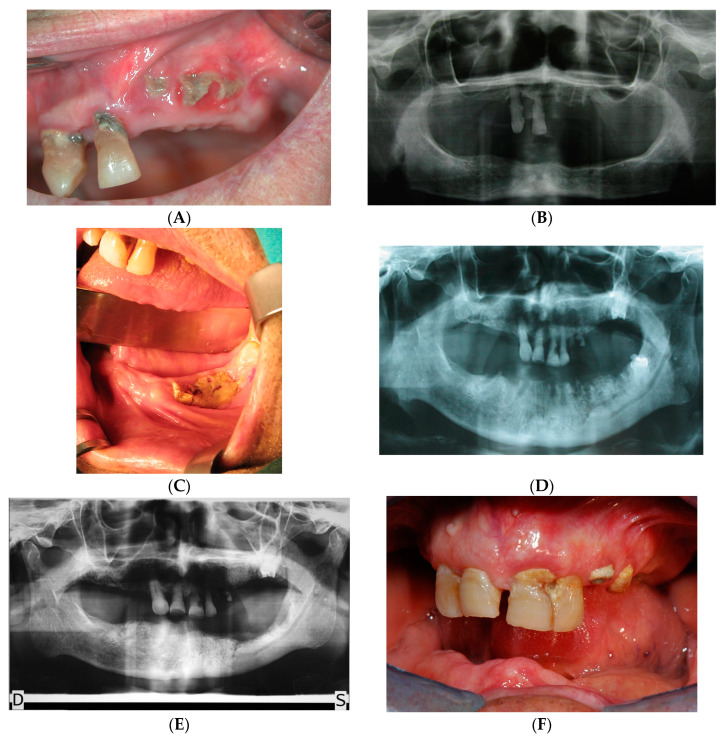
Clinical and radiological examinations. (A) Female patient 89 years old Stage 1 AAOMS. Necrotic exposed bone in the upper left jaw with poor oral hygiene status. (B) Orthopantomogram of the same patient. (C) Male patient 80 years old. Stage 1 AAOMS. (D) Orthopantomogram of the same patient. (E) Orthopantomogram after surgical ablation. D, right. S, left. (F) Post-surgical plaque control remained unsatisfactory.
